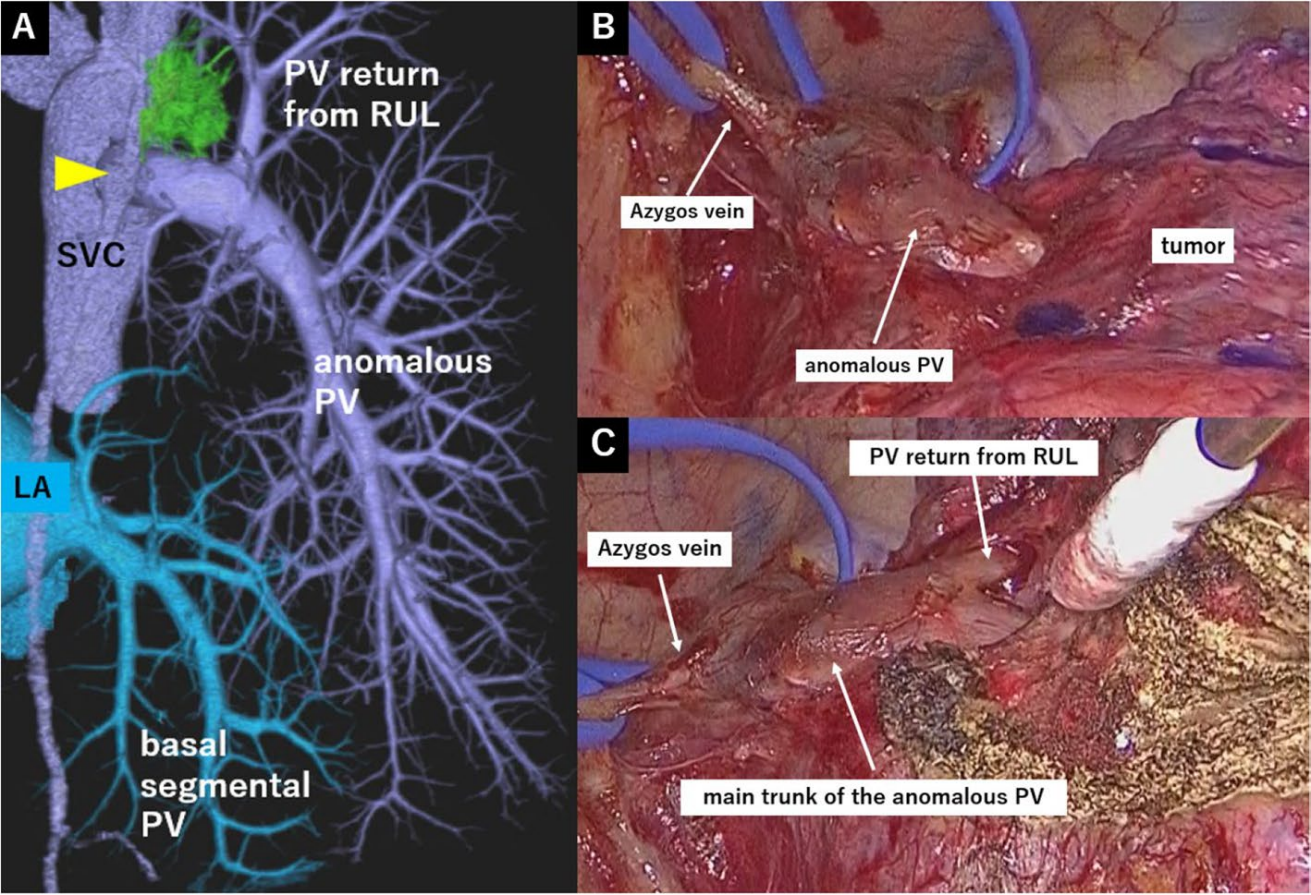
A 3D-CT Angiography. Except for only a few basal segmental PV, most pulmonary veins returning to the azygos vein (arrow). B Perioperative image. Anomolous PV drained into the azygos vein. C The tumor is located closer to the main trunk of the anomalous PV than to the PV return from RUL
